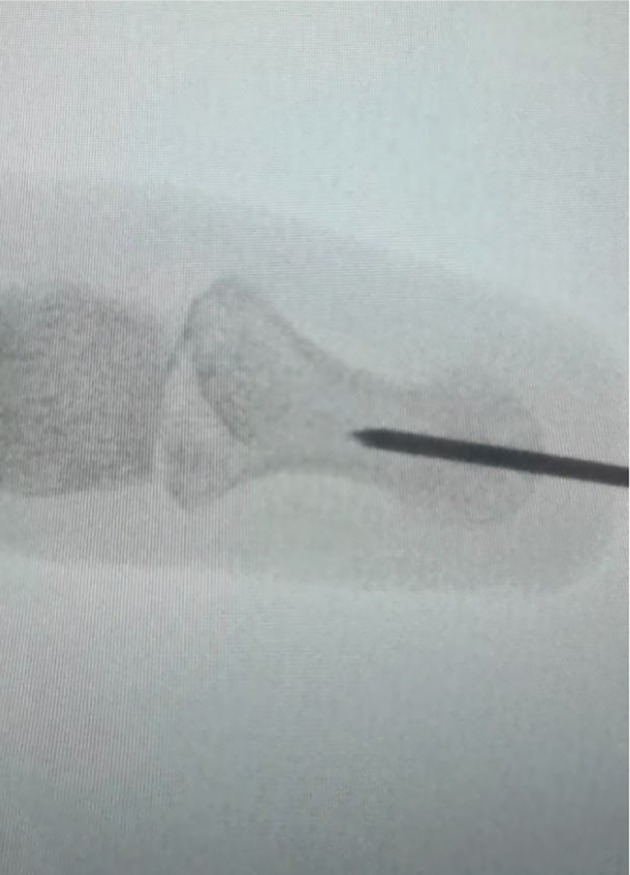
Intraoperative radiograph showing retrograde drilling of a 1.1 mm K‐wire through the tuft towards the joint surface.
